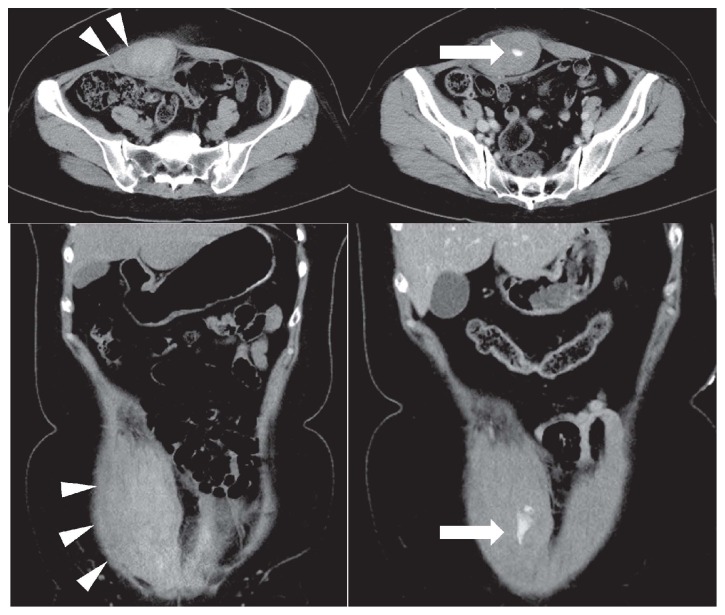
Abdominal and pelvic computed tomography demonstrated bilateral rectus sheath hematoma (white arrowhead). The hematoma extended into the median umbilical fold, forming a large mass that spread to the suprapubic area.
